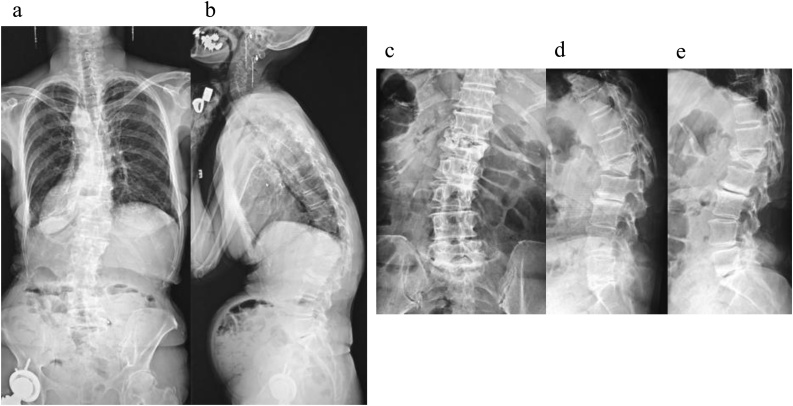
Preoperative radiographs of a 68-year-old female patient with OVF. Preoperative radiographs of a 68-year-old female showing kyphoscoliotic deformity at the thoracolumbar spine associated with non-union of OVF at L1. (a) Standing whole-spinopelvic AP radiograph, (b) Standing whole-spinopelvic lateral radiograph, (c) Lumbar AP radiograph, (d) Lumbar lateral radiograph in flexion, and (e) Lumbar lateral radiograph in extension.
